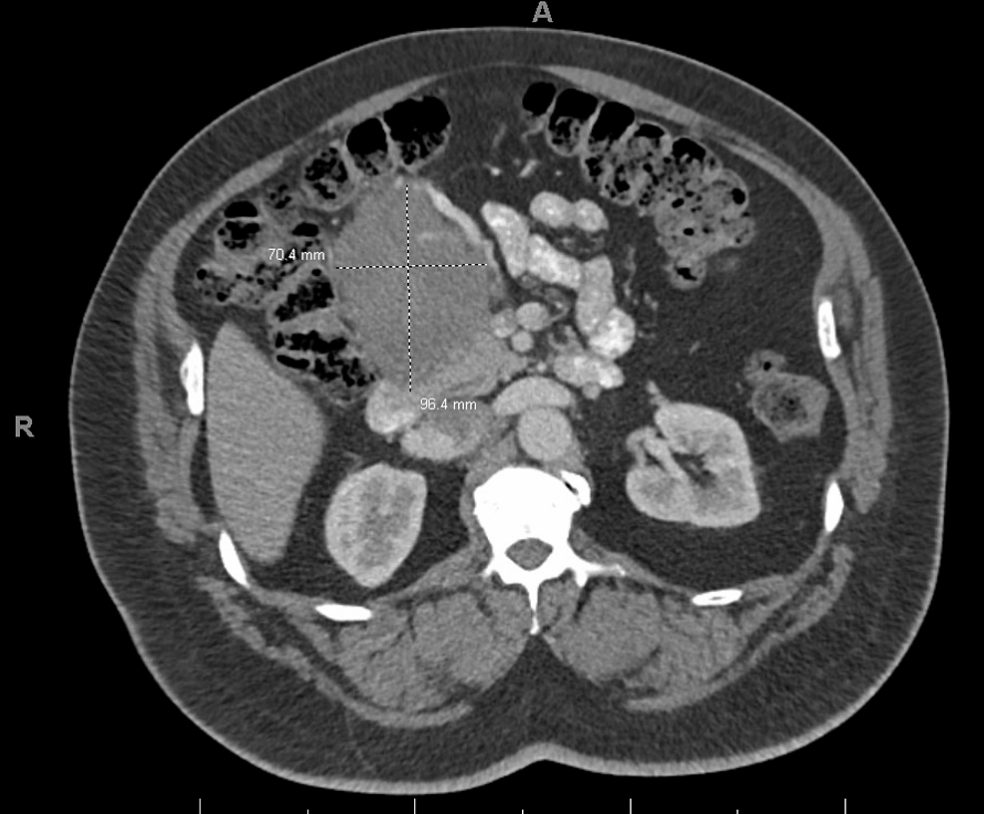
CT abdomen and pelvis following chemotherapy shows a reduction in the size of the duodenal mass to 9.6 x 7.0 x 7.2 cm.
